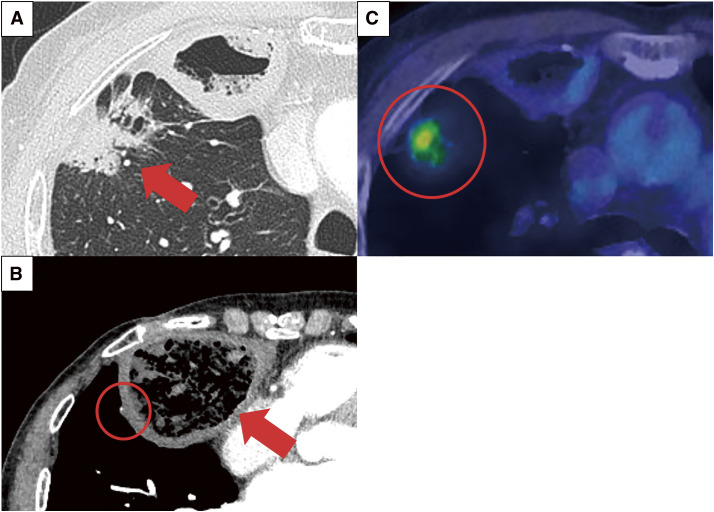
(A) Preoperative CT scan showing the tumor, measuring 43 mm at its largest dimension, located in the anterior segment of the right upper lobe (red arrow). (B) The reconstructed gastric tube protruding into the right thoracic cavity, positioned close to the right lung (red arrow) and its feeding vessel (red circle). (C) ^18^F-FDG positron emission tomography/CT demonstrating FDG uptake in the tumor with a maximum standardized uptake value of 4.0 (red circle). CT, computed tomography; FDG, fluorodeoxyglucose
